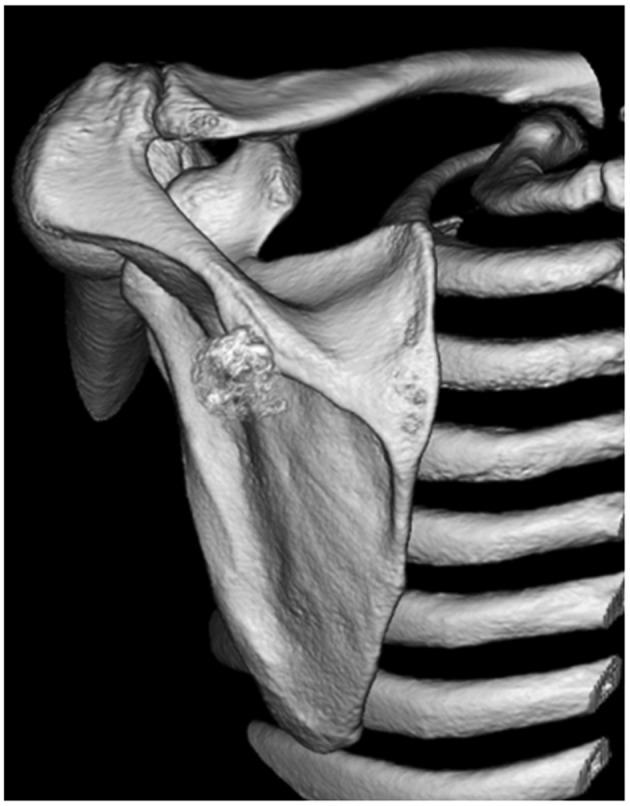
Three-dimensional computed tomography (CT) image showing the presence of a lesion and the normal appearance of the scapula.
